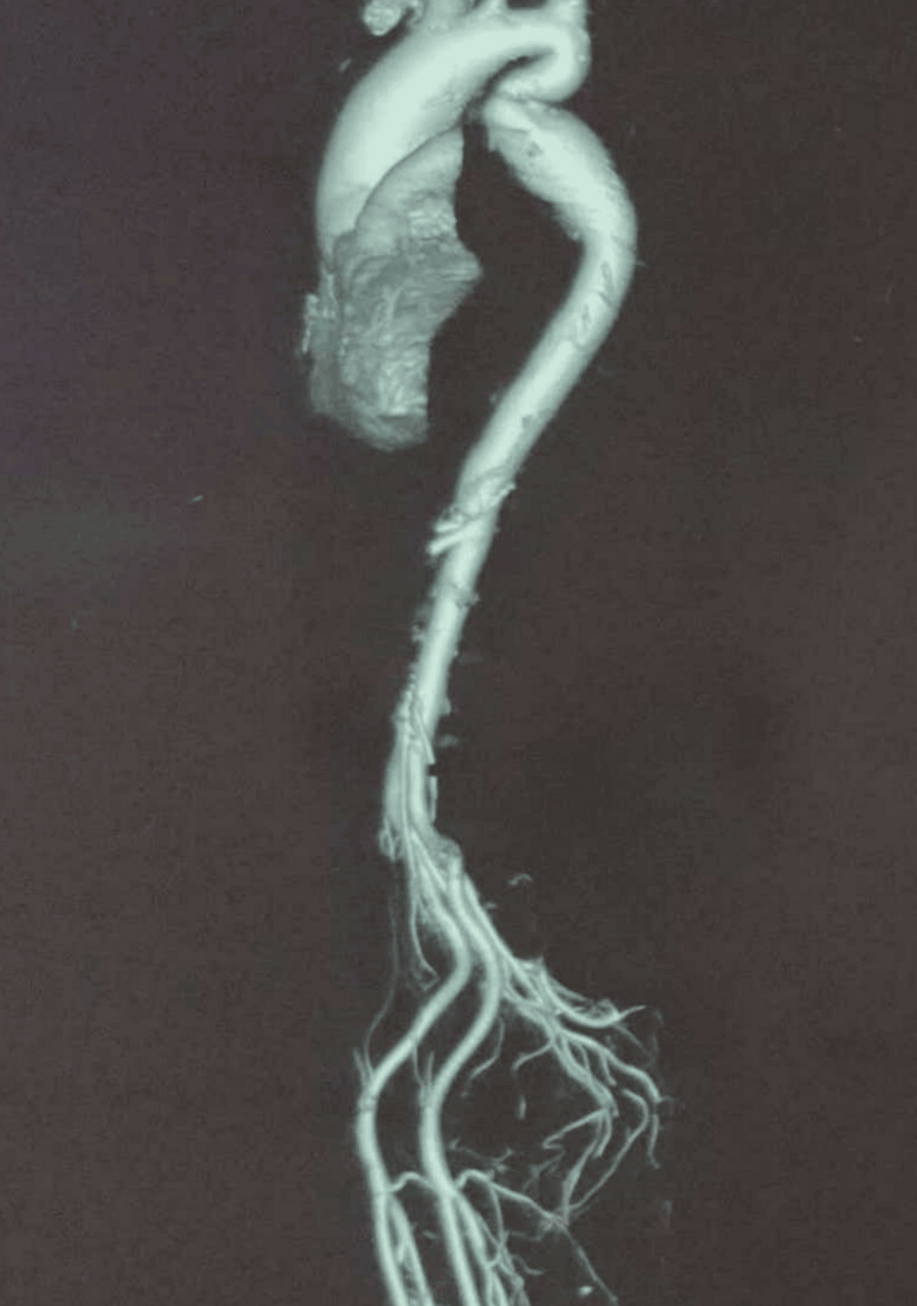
CT aortogram Fusiform aneurysm of aortic root with kinking of the descending thoracic aorta and absence of collateral circulation. CT: computed tomography
